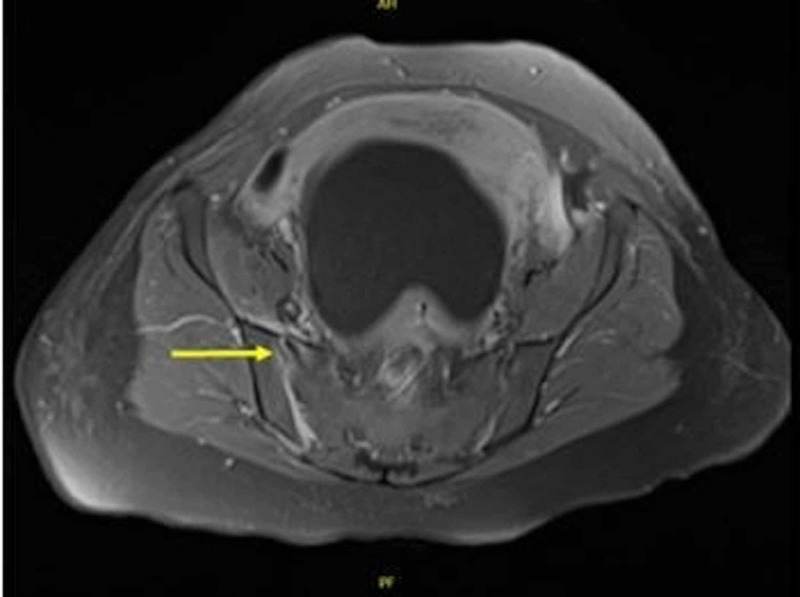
MRI pelvis demonstrating edematous changes (arrow) of the right sacroiliac (SI) joint, indicative of septic sacroiliitis
